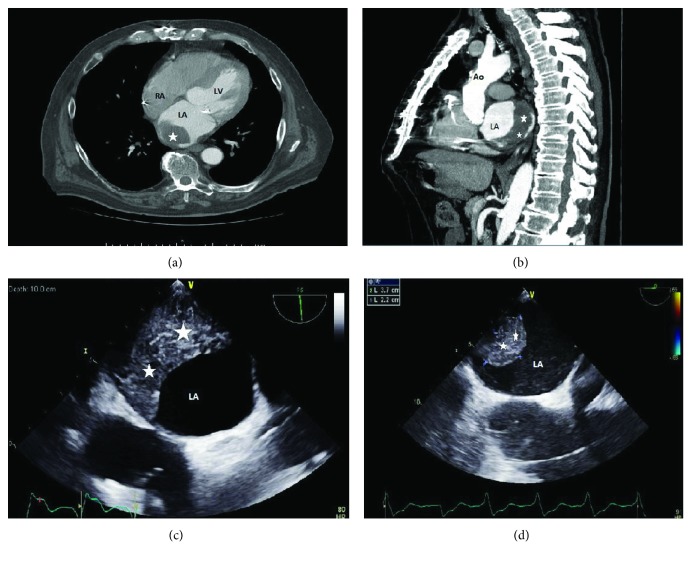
(a and b) Computed tomography (CT) of the chest showing a thrombus (stars) in the left atrium of the heart (LA). (c and d) Transesophageal echocardiogram (TEE) showing the thrombus in the left atrium of the heart (LA). Ao, aorta; LA, left atrium; LV, left ventricle; and RA: right atrium.
